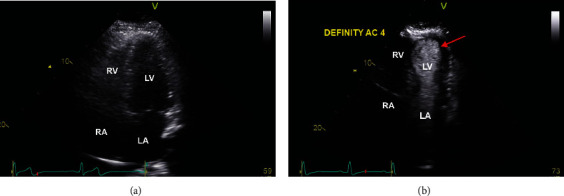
Transthoracic echocardiogram, apical 4-chamber view, demonstrates (a) normal LV contractility three years prior and (b) contrasted study with LV dysfunction with apical dyskinesia (red arrow), consistent with takotsubo cardiomyopathy.
